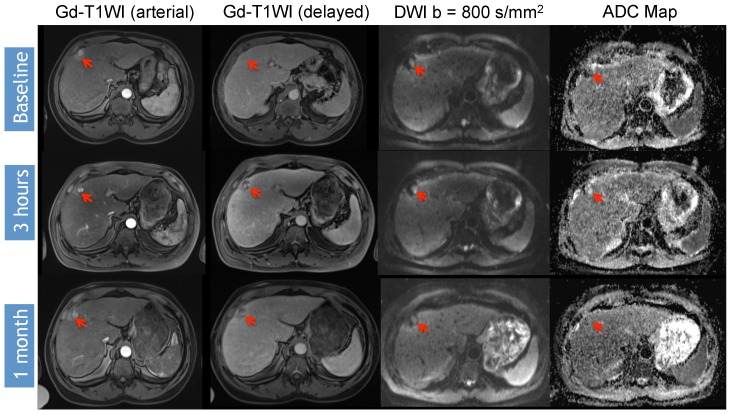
DWI Response Assessment after DEB-TACE treatment. 69-year-old man presented with a segment V HCC (arrow) without macrovascular invasion or extrahepatic disease. Note that there is decreased peripheral enhancement at 3 h that becomes more evident at one month. When analyzing DWI images, ADC values increased ≥20% in the treated region (baseline ADC 0.704 × 10^−3^ mm^2^/s, post-3 h ADC 1.30 × 10^−3^ mm^2^/s); however, a small nodule of restricted diffusion persists at one month (ADC 0.940 × 10^−3^ mm^2^/s). The findings represent a subjective and objective measurement of response, in this case representing partial response (PR) to therapy.
